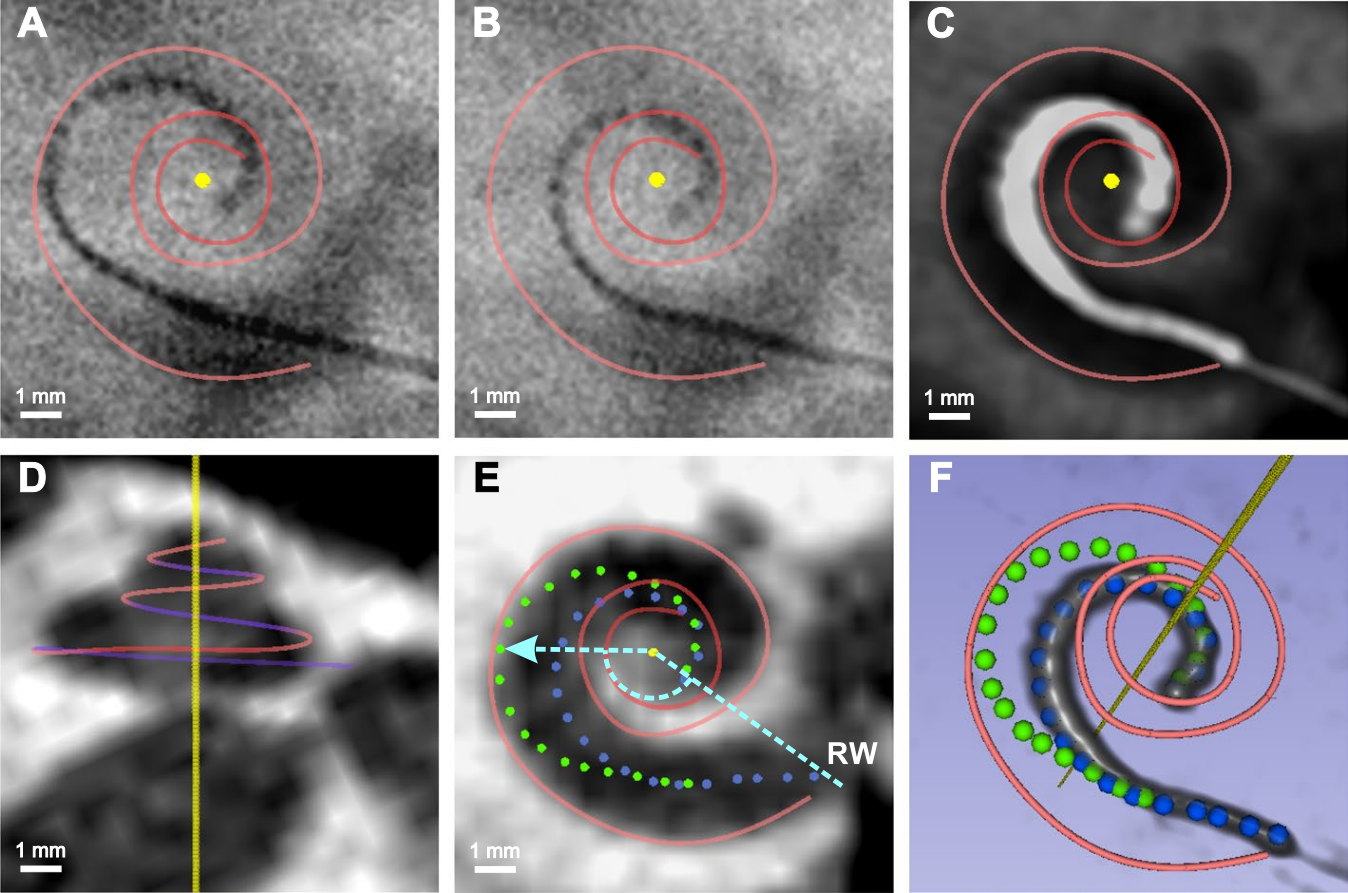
Overview of imaging techniques, registration, and measurements. Example of the fluoroscopy at maximal insertion (A) and pull-back (B) positions with reconstruction of outer wall spline (red) and mid-modiolar axis (yellow dot). Preoperative (D, E) and postoperative (C) CBCT images with indication of the scala tympani outer wall (red spline) mid-modiolar axis (yellow) and round window (RW). An example measurement of insertion angle and distance is indicated with a dashed cyan line. (F) 3D reconstruction of the final electrode position with scala tympani outer wall (red spline), calculated mid-modiolar axis (yellow axis), and individual electrode locations at maximal insertion (green dots) and pull-back (blue dots) positions. The blue markers overlap with the actual position of the cochlear implant.
